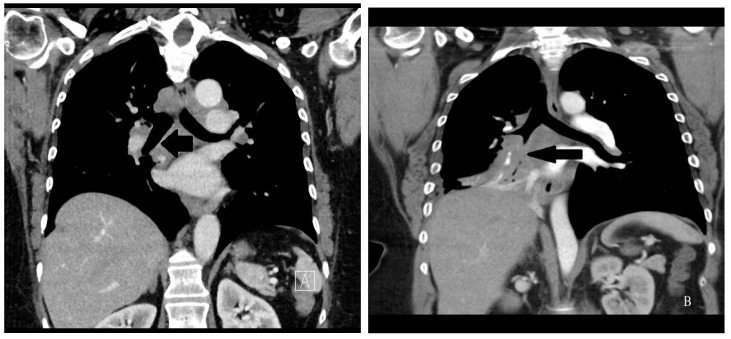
(A) Left figure: Chest CT sagittal image of mediastinal lymph nodes from sarcoidosis. The lymph nodes do not appreciably disturb airway patency, and the right bronchus intermedius is completely unobstructed (arrow); (B) Right figure: Chest CT sagittal image of mediastinal lymph nodes from small cell lung carcinoma. The lymph nodes do not respect anatomic boundaries and compromise the right bronchus intermedius (arrow).
